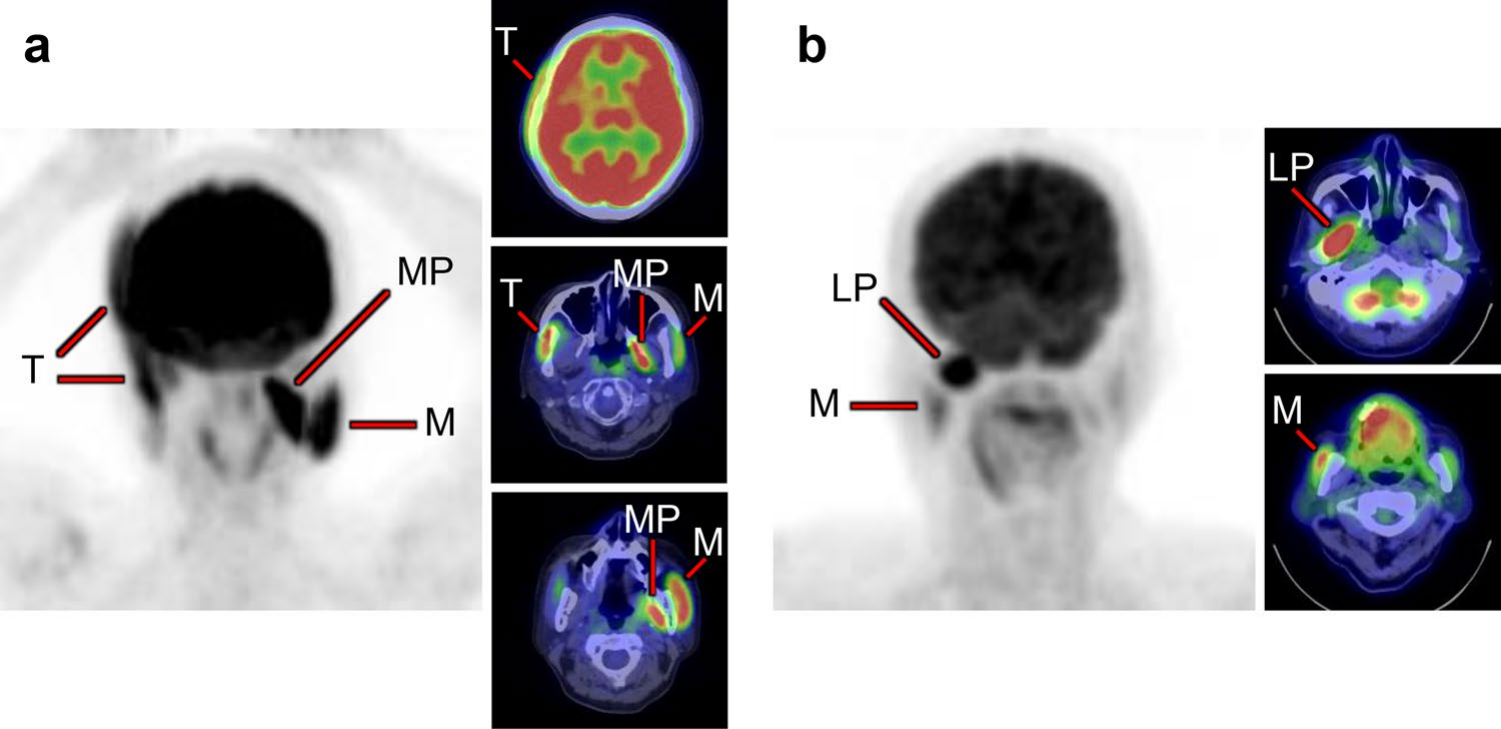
Muscles of mastication.* Increased [^18^F]FDG uptake in masticatory muscles, consisting of the masseter (M), temporalis (T), medial pterygoid (MP), and lateral pterygoid (LP), is well known to be observed when chewing gum, but there are also various causes such as involuntary movements and bruxism. A few masticatory muscles are often depicted at the same time, and rarely are they seen alone. They are usually visualized as asymmetrical. A case with increased [^18^F]FDG uptake at the right temporalis, left masseter, and left medial pterygoid (a), and a case with uptake at the right lateral pterygoid and right masseter (b) are shown
